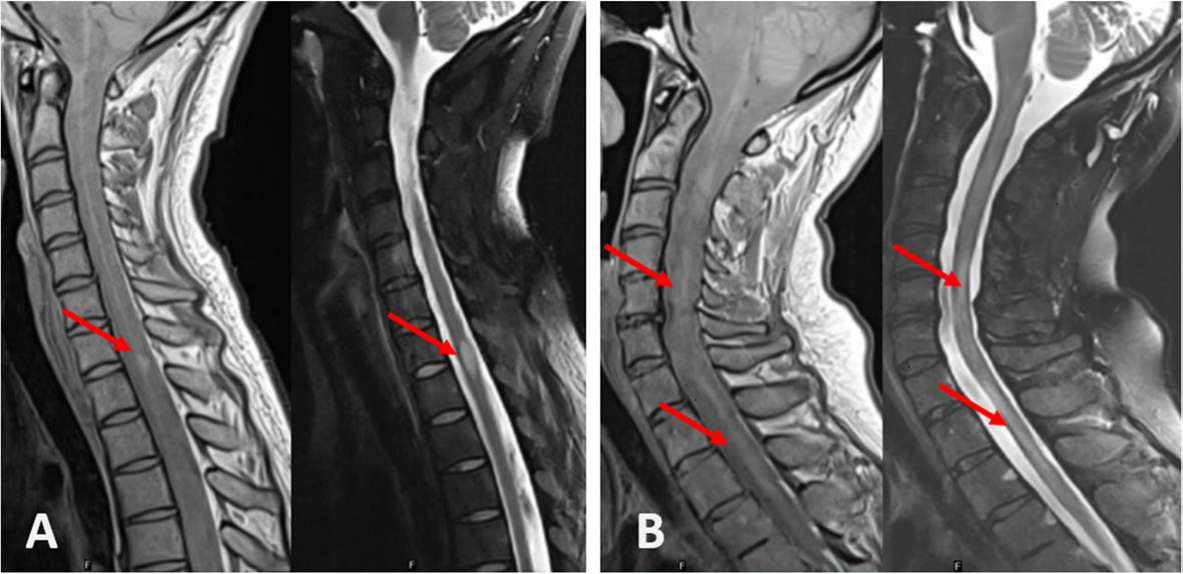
Examples of focal and diffuse spinal cord lesions (left PD sequence and right T2W sequence) on diagnostic MRI of the cervical and upper thoracal spinal cord in two patients with MS. A: focal lesion without diffuse lesions in a 24-year-old patient (EDSS 2.0) B: diffusely abnormal signal without clearly demarcated focal lesions in a 40-year-old patient (EDSS 3.5)
